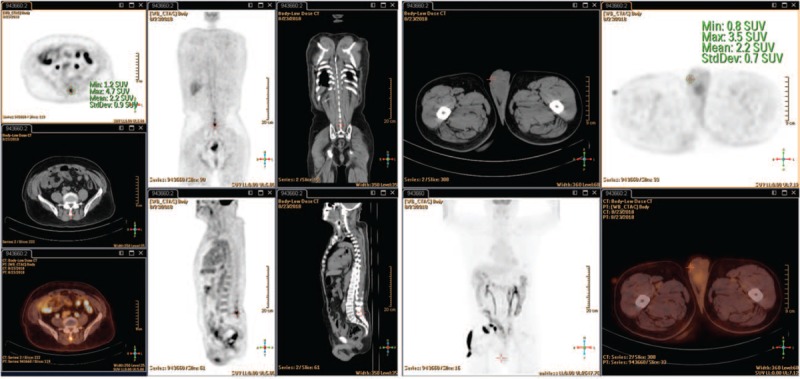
Positron emission tomography with fluorine-18 fluorodeoxyglucose integrated with computed tomography showed no evidence of distant metastases and locoregional failure of the urothelial carcinoma.
